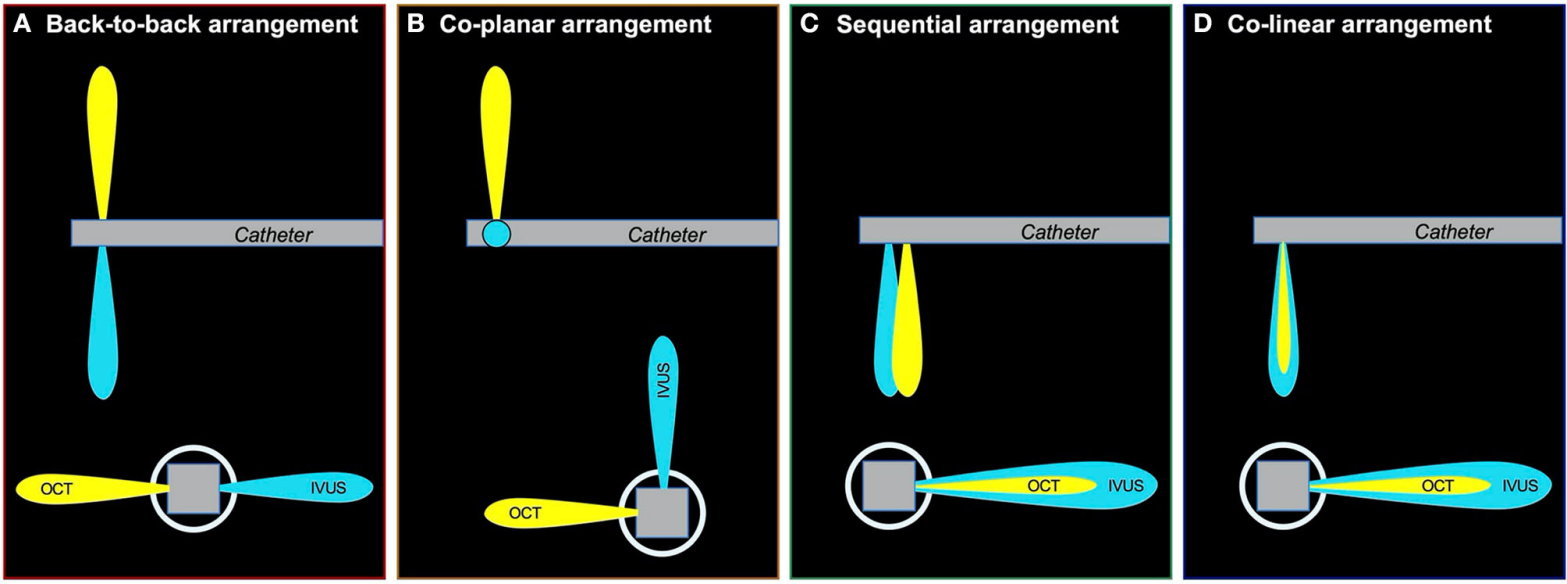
Various types of arrangements of IVUS and OCT transducer. There were three types of arrangements of IVUS and OCT transducer; (A): back-to-back arrangement; (B): coplanar arrangement; (C): sequential arrangement; (D): colinear arrangement. The colinear arrangement can acquire the strictest coregistration image of IVUS and OCT in three types of arrangements. IVUS, intravascular ultrasound; OCT, optical coherence tomography.
